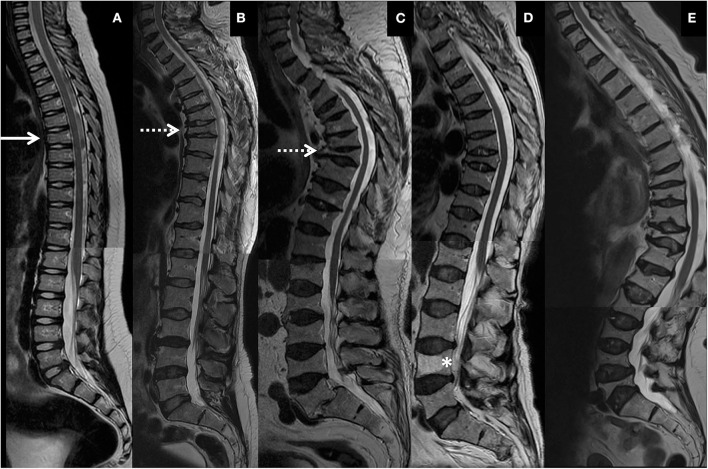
Representative spinal magnetic resonance images of thoracic and lumbar spines of four *PLS3* mutation-positive subjects. (A) 9-year-old male, (B) 46-year-old male, (C) 77-year-old male, and (D) 70-year-old female. Images show mild vertebral changes in childhood (solid arrow), multiple and severe vertebral compression fractures and kyphosis in early adulthood (dotted arrows), progression of spinal changes with increasing age. (D) portrays vertebral changes in heterozygous females and a vertebral hemangioma (asterisk). Subject in (A) harbors an intragenic tandem duplication within *PLS3*, and subjects in (B–D) a heterozygous/hemizygous *PLS3* deletion c.73–24T>A (p.Asp25Alafs*17). (E) Shows thoracic and lumbar spine images of a 74-year-old female with WNT1 osteoporosis due to a heterozygous *WNT1* mutation p.Cys218Gly, portraying the differences between PLS3 and WNT1 osteoporosis in type and location of vertebral compression fractures, changes in spinal stature and presence of simultaneous deterioration in cartilaginous tissues. The images have been combined from separate MR-images of thoracic and lumbar spines.
